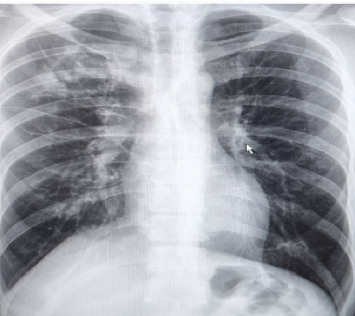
The patient's chest X-ray.
